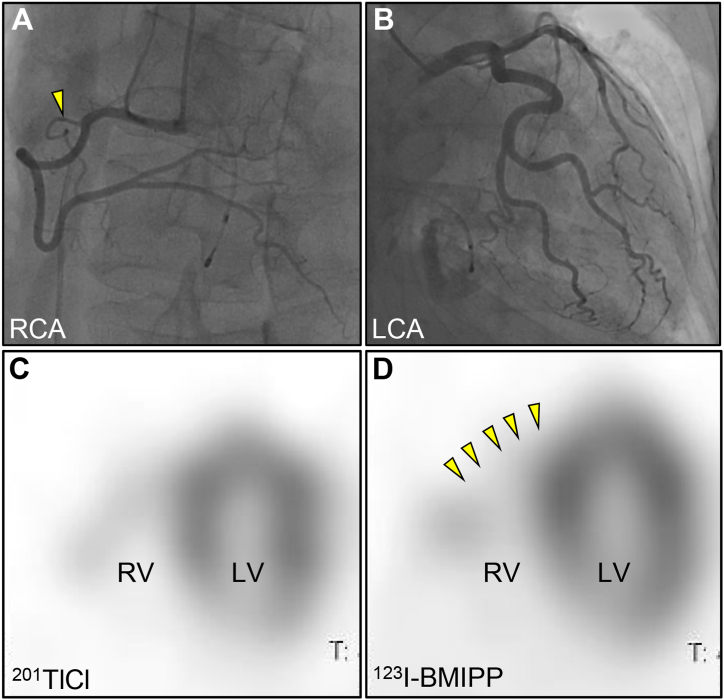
Coronary angiography and dual-isotope myocardial SPECT Coronary angiography was unremarkable including a right ventricular branch (arrowhead) (A, B). Myocardial ^201^TlCl and ^123^I-BMIPP SPECT images (long axis vertical view) (C, D). Myocardial perfusion of 201TlCl is preserved, whereas 123I-BMIPP is reduced in the apico-mid region of the RV (arrowheads), corresponding to the wall motion abnormalities identified on initial echocardiography. BMIPP, (β-methyl-*p*-Iodophenyl-Pentadecanoic Acid); LCA, left coronary angiography; LV, left ventricle; RCA, right coronary angiography; RV, right ventricle; SPECT, single-photon emission computed tomography.
